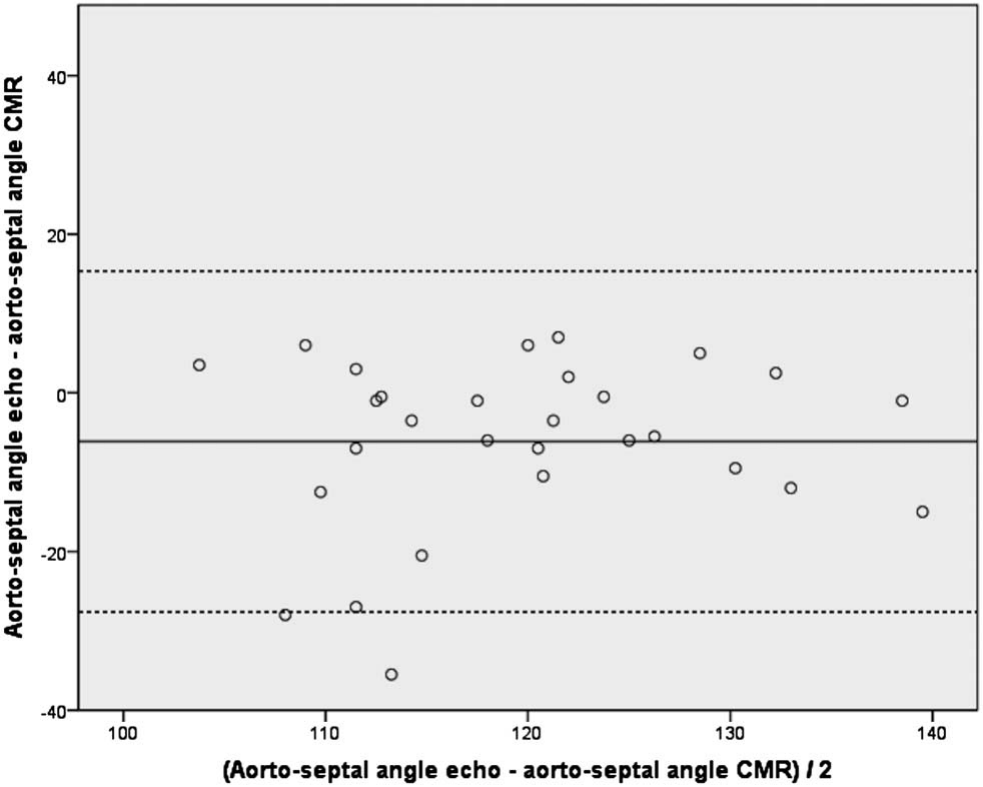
Bland-Altman plot of the differences between aortoseptal angulation measured using transthoracic echocardiography and cardiac MR (CMR) imaging. Solid line represents mean, dashed line represents mean ±2 SDs.
